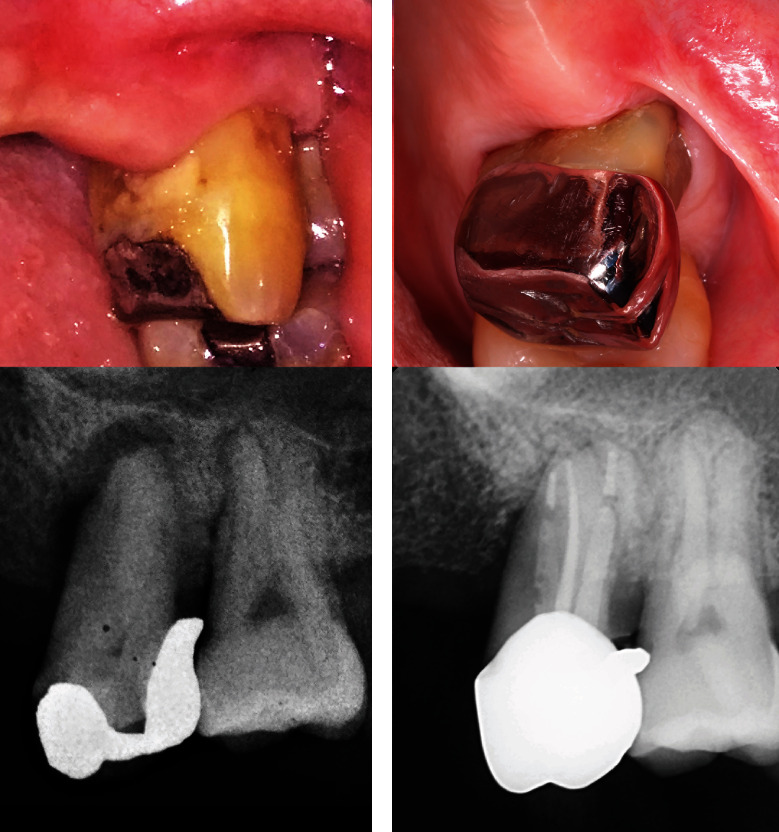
Comparison between (a) initial and (b) final clinical and radiographic condition.
